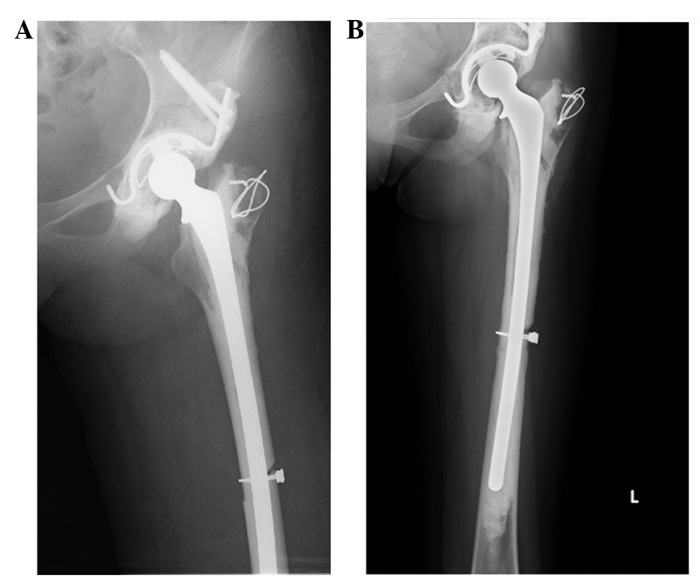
Radiographic observations following the second surgery. (A) Acetabular side and (B) femoral side. The acetabular side has been reconstructed using the pasteurized bone, a Kerboull-type (KT) plate and an acetabular cup with bone cement. The femoral side has been reconstructed using the long stem with bone cement.
